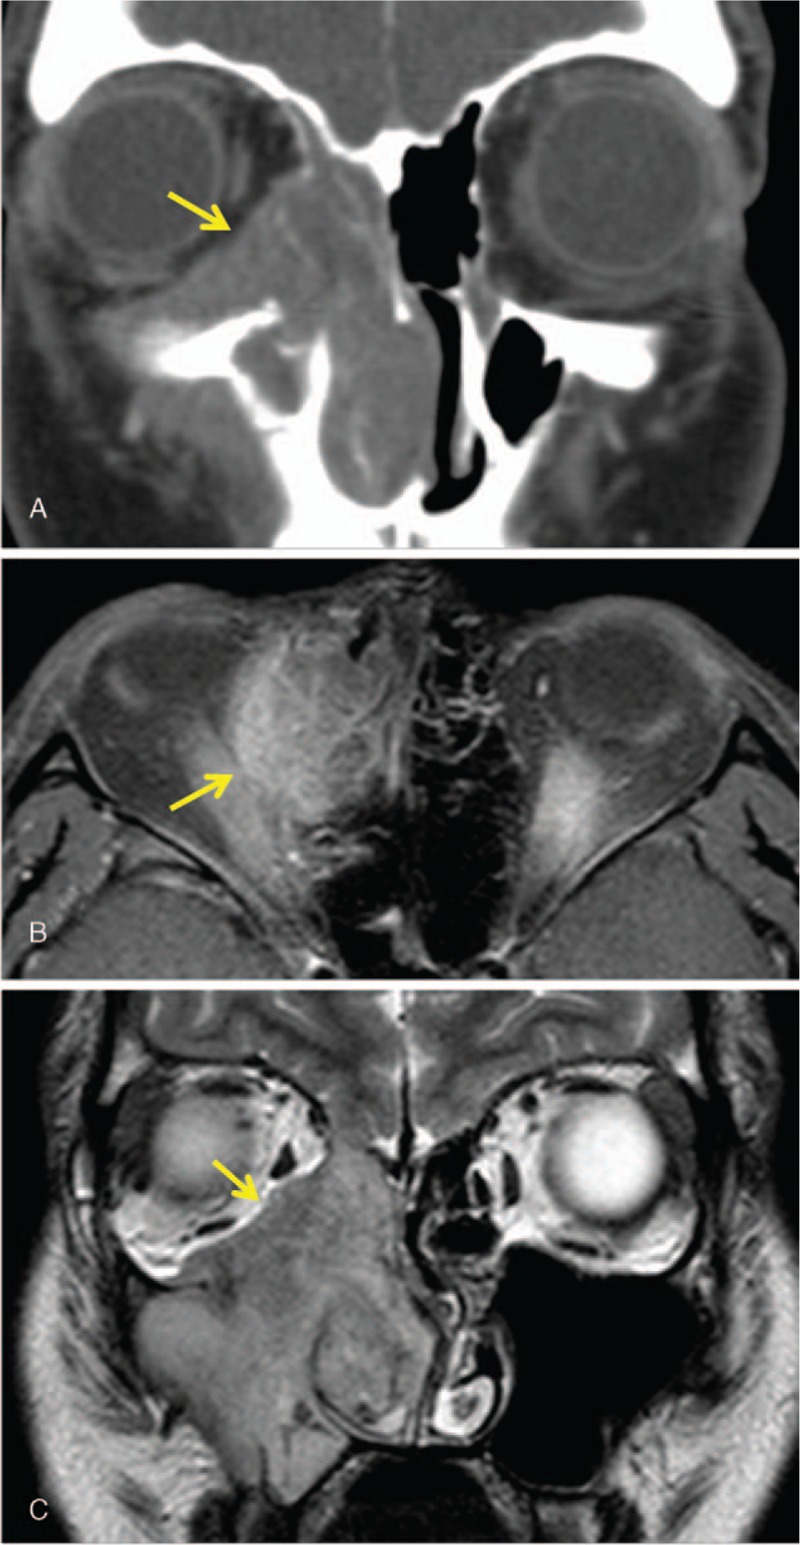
Coronal computed tomography scan (A), axial (B) and coronal (C) magnetic resonance imaging showing a large homogenous well-enhanced mass (6.4 × 4.7 cm) with surrounding bony erosion and remodeling.
